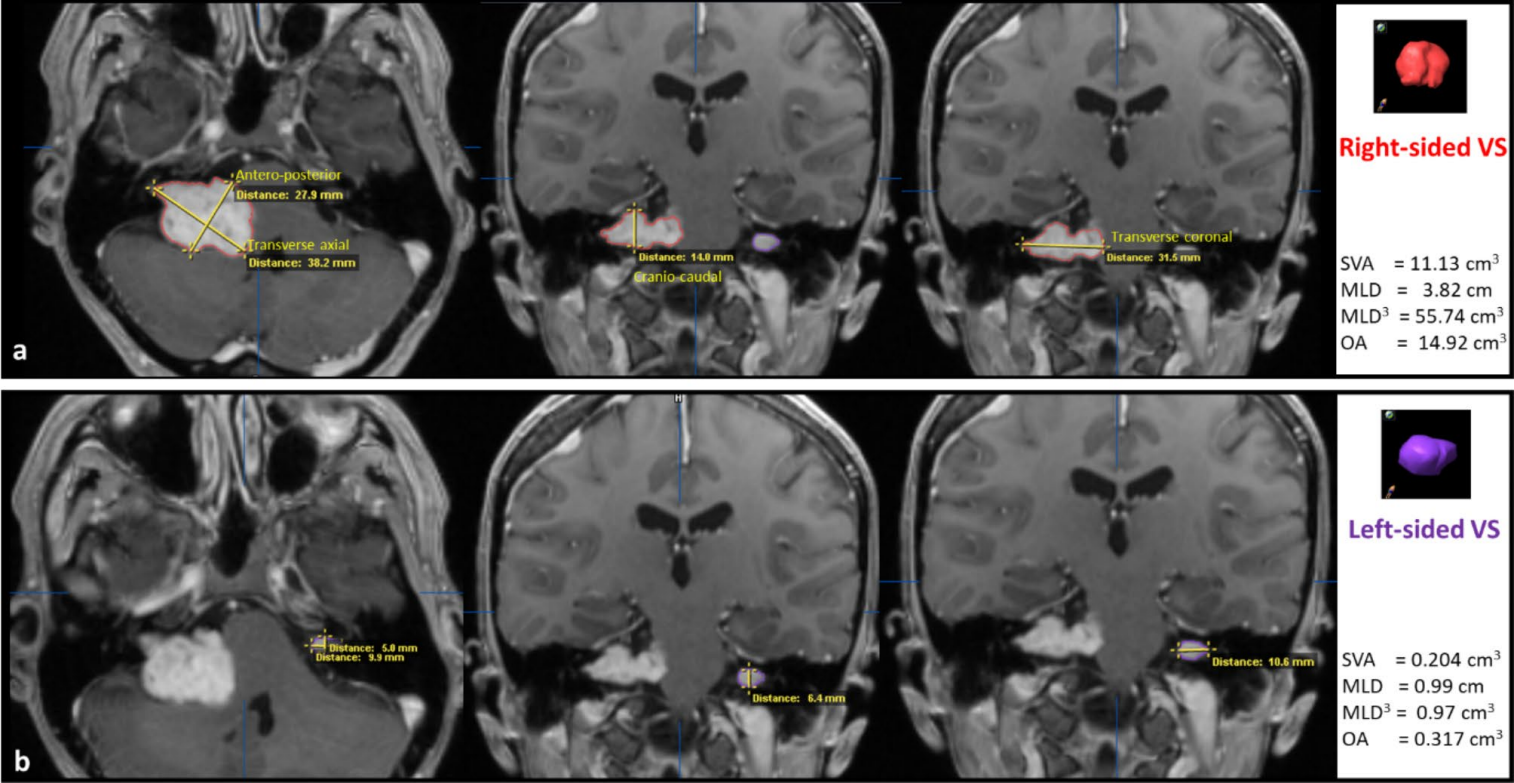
Segmented volumetric analysis (SVA) and linear measurements in (a) a right- and (b) left-sided NF2-associated vestibular schwannoma. T1-weighted contrast-enhanced, thin-sliced (1 mm) MRI in axial (left) and coronal (middle and right) planes. In the SVA (iPlan Net Software, Brainlab, semiautomated and manual segmentation), the external contours of the right (red color) and left (violet color) are traced, and 3D-volume is assessed (3D-model on the right side in the appropriate tumor color. Tumor diameters were measured in anteroposterior, transverse, and craniocaudal dimensions in axial and coronal planes (defined as “T1 ax width” = anteroposterior and “T1 ax width” = transverse tumor diameter in the axial plane, and “T1 cor width” = craniocaudal tumor diameter in the coronal plane). The greatest linear measurement was defined as the Maximum Linear Diameter (MLD), which was additionally cubed for the volumetric analysis. The maximum linear measurements in the anteroposterior (transverse), cranio-caudal (coronal), and transverse (axial plane) were multiplied to give a second volumetric estimate in the Orthogonal Analysis (OA). The MLD and OA were defined and calculated similarly to previous studies for better comparison. In addition, we have included a fourth dimension (defined as “T1 cor length” = transverse tumor diameter in coronal planes) separately to gain a comprehensive impression.
